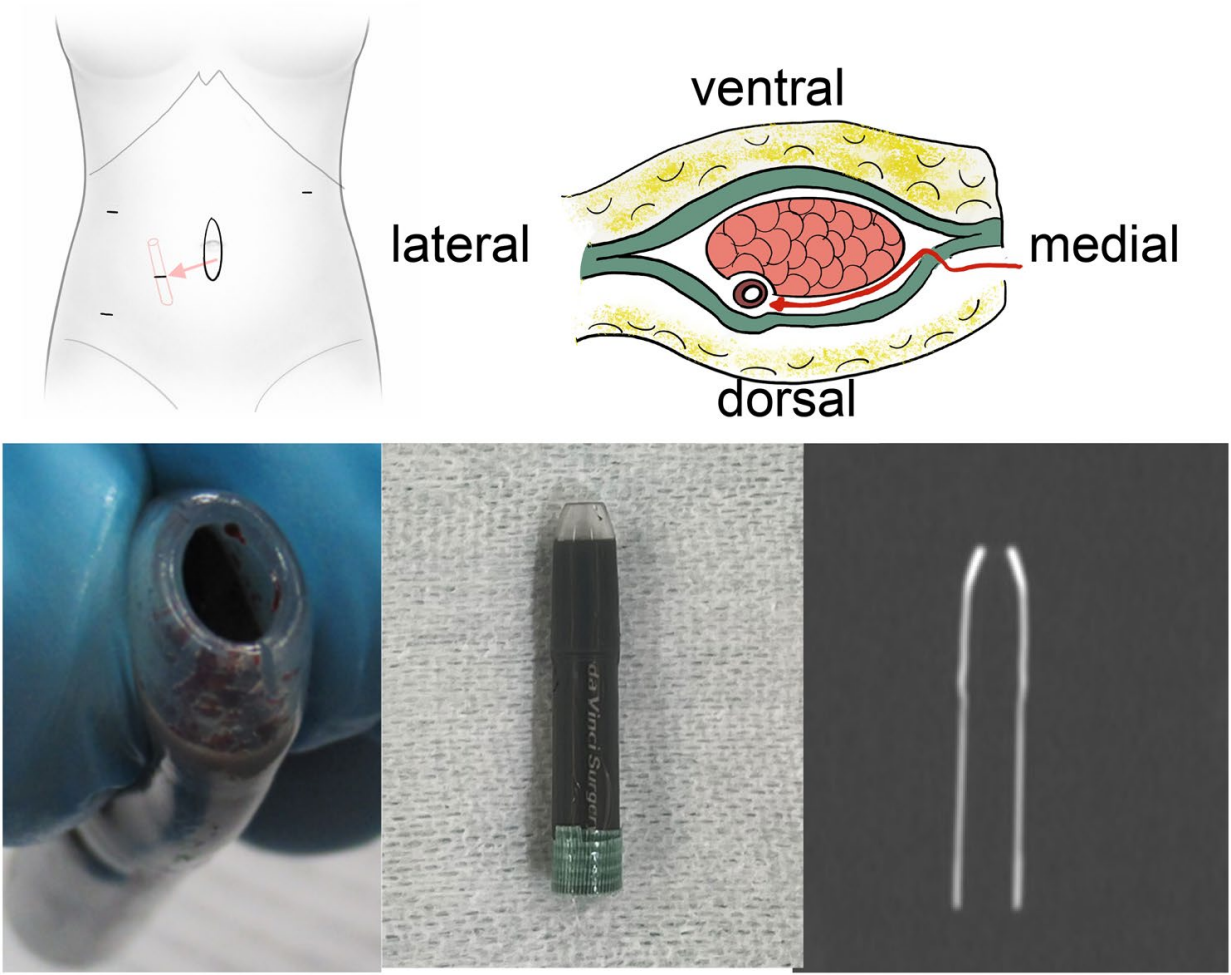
Localization and retrieval of the retained robotic tip cover with corresponding CT characteristics. The retained tip cover was approached through a midline incision (upper left part). After opening the posterior rectus sheath, the object was accessed from the dorsal side of the rectus abdominis muscle and retrieved (upper right part). The extracted tip cover showed a cylindrical hollow interior and consisted of three distinct components. Retrospective CT analysis demonstrated attenuation values of +150, −300, and −430 Hounsfield units, from the distal end proximally (lower part).
